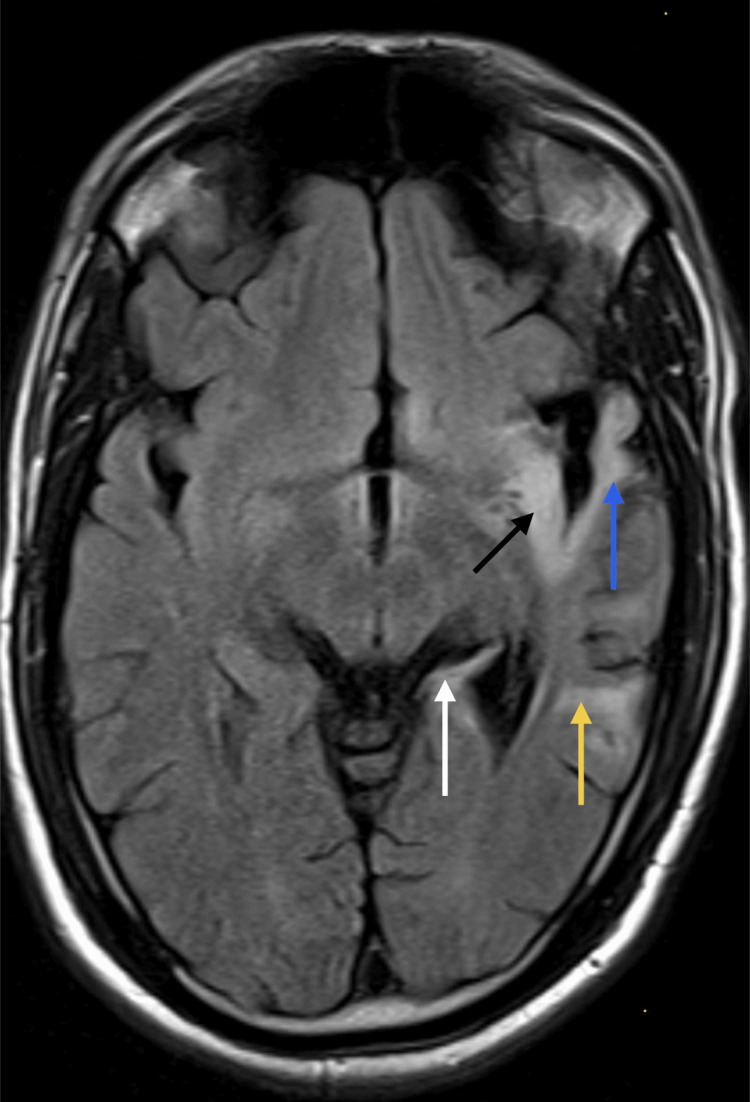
MRI brain axial FLAIR image taken on January 9, 2024, showing hyperintensity involving the left insula (black arrow), temporal operculum (blue arrow), lateral temporal lobe (yellow arrow), posterior hippocampus (white arrow), and bilateral medial orbital cortex. FLAIR: Fluid-attenuated inversion recovery.
